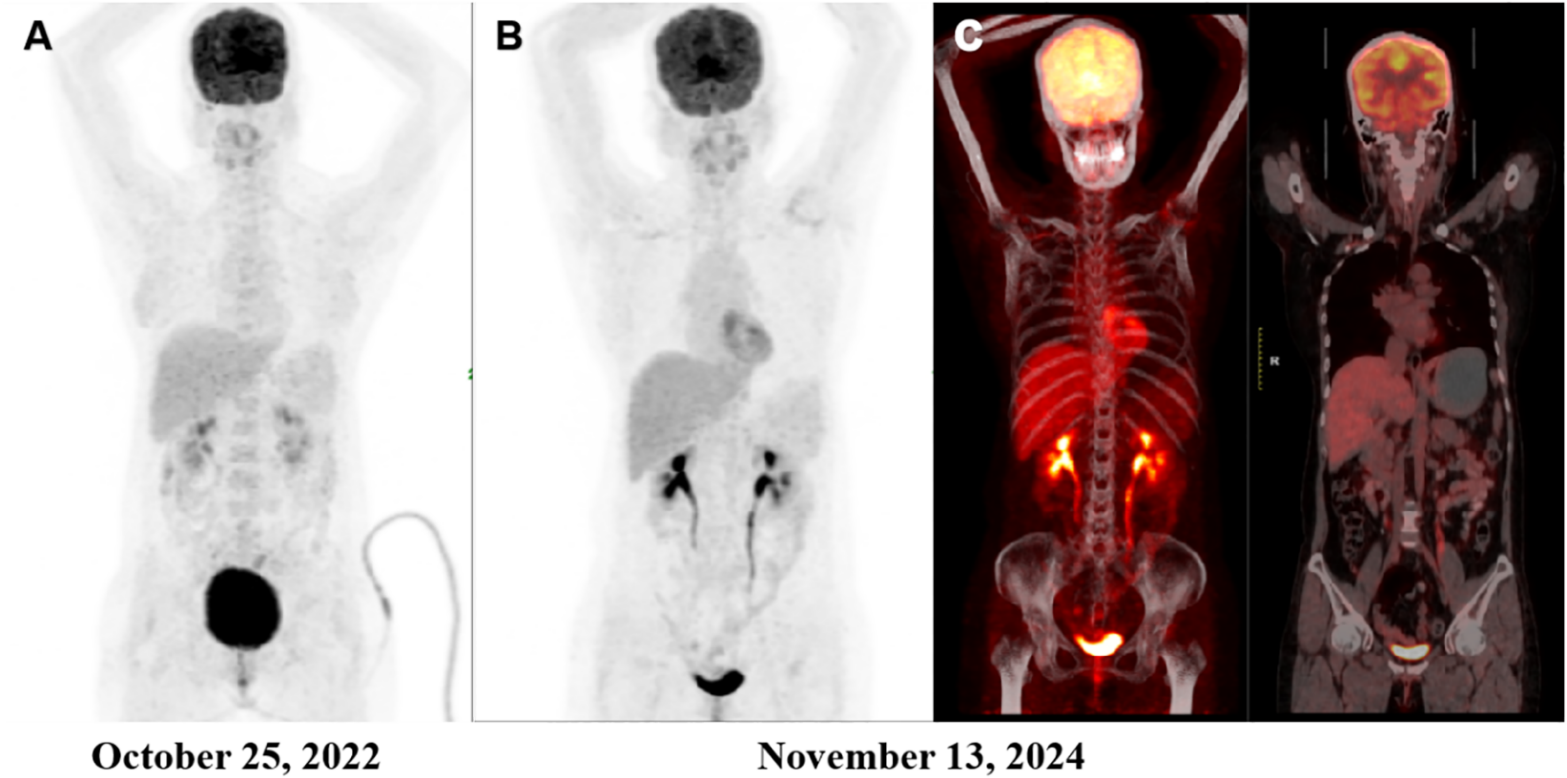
Comparison of PET/CT scans before and after treatment. (A) Preoperative PET/CT image showing no hypermetabolic activity in the liver. A 1.6 cm cystic lesion was noted in the right adnexal region, and a physiologically active focus was observed in the left adnexal region (SUVmax = 4.5). (B, C) Post-treatment PET/CT images obtained 19 months after surgery revealed no abnormal hypermetabolic activity suggestive of recurrence.
